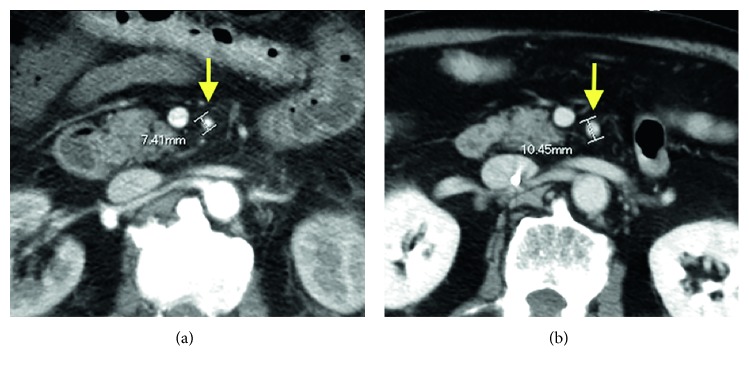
Coronal-section contrast-enhanced CT of the abdomen. (a) Before surgery. (b) 3 months after surgery. The diameter of the superior mesenteric artery is 7.41 mm before surgery and 10.45 mm at 3 months after surgery (arrows).
